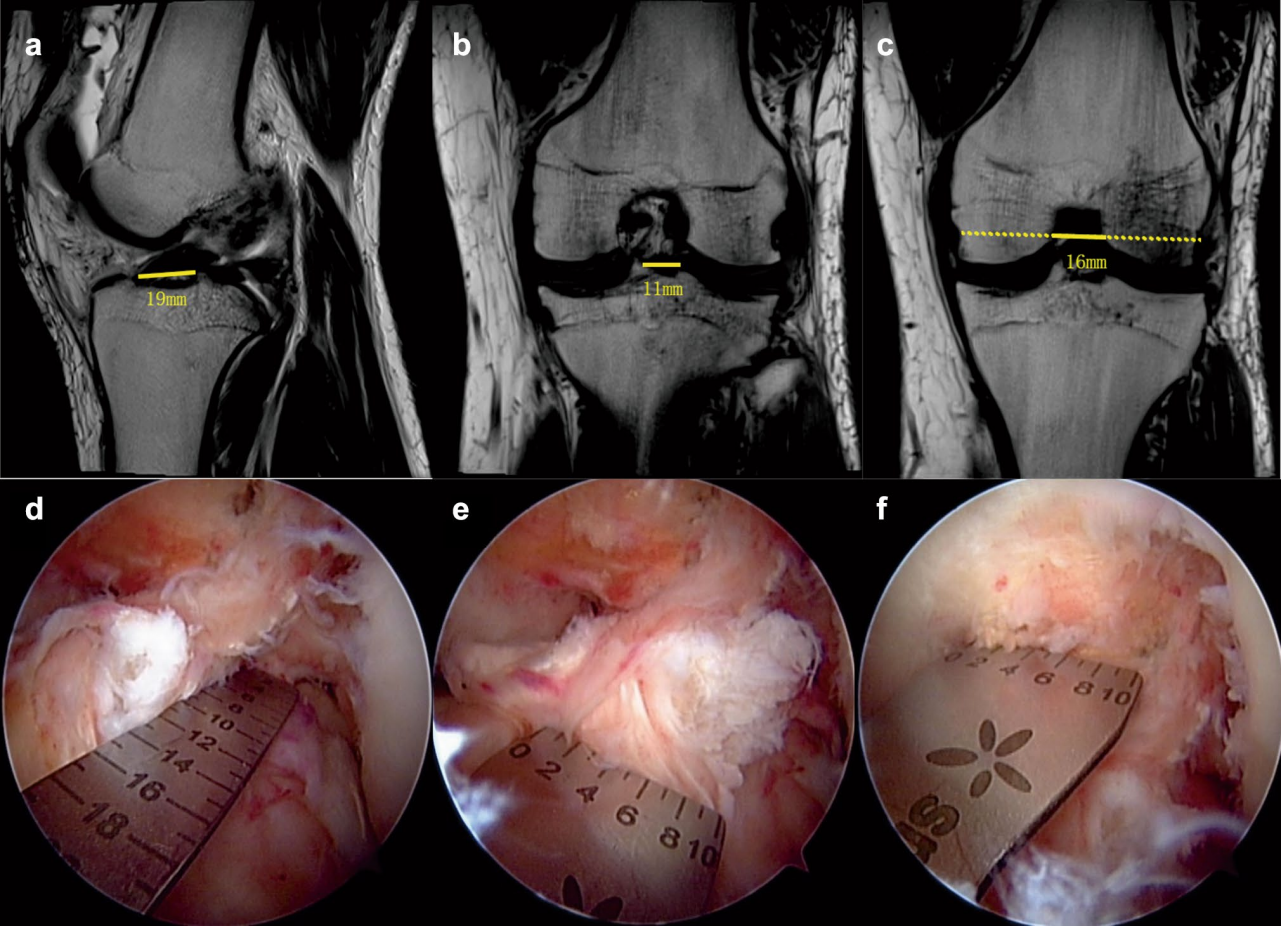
The nether pictures are arthroscopic views of a knee in flexion. The length (d) and width (e) of the notch (f) are measured using an arthroscopic ruler. A T2 proton density-weighted sequence of knee MRI showing a rupture of the ACL and the knee intercondylar notch. a The yellow line signifies the tibial ACL insertion site length. b The yellow line signifies the tibial ACL insertion site width. c The full yellow line signifies the knee intercondylar notch width
